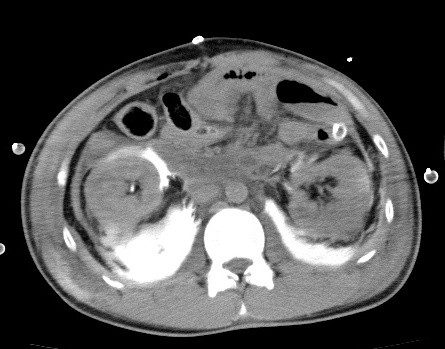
Abdominal CT scan at 9 hours after surgery shows contrast retention in the perirenal retroperitoneum bilaterally.
